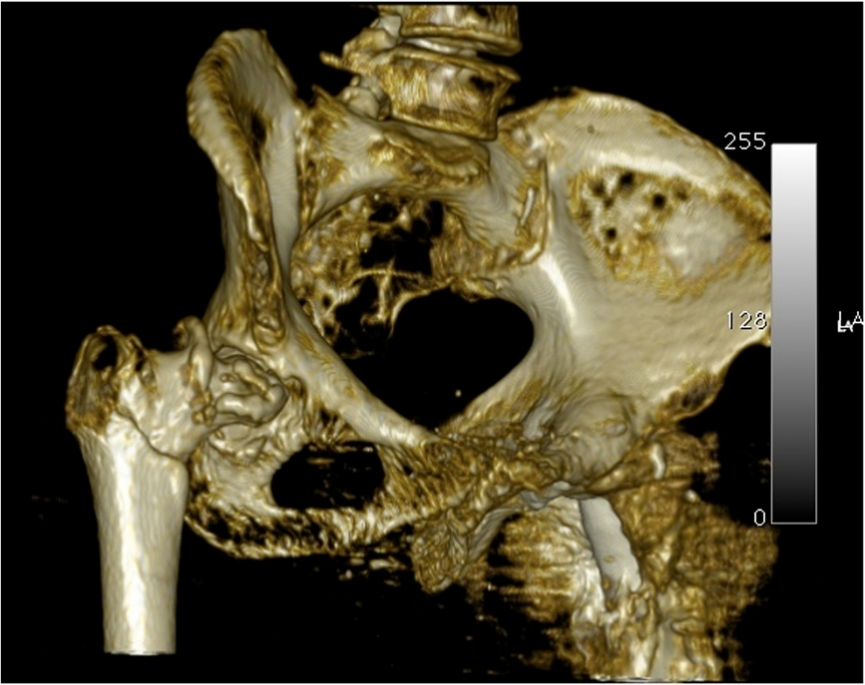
Three-dimensional computed tomography scan of her right hip showing a simple intertrochanteric fracture associated with femoral head stage VI avascular necrosis, with the femoral head impacted in the acetabular rim.
